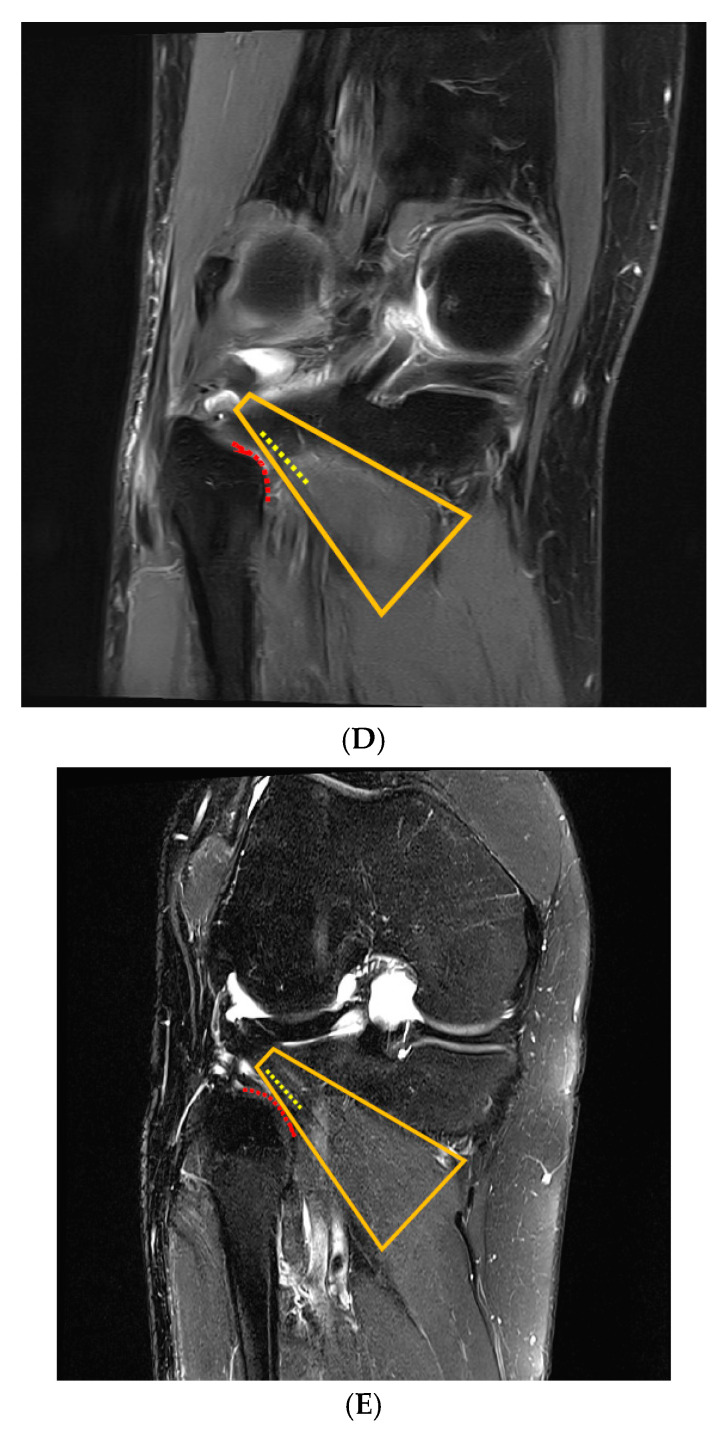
(A) An axial image from the postoperative CT scan. On CT scans with the knee in full extension, the osteotomy plane (yellow dotted box) adjacent to the proximal tibiofibular joint is located on the more medial side parallel to the proximal tibiofibular joint. TSO: tibial-sided osteotomy. (B) On the axial MRI of the knee in full extension, the TSO plane (yellow dotted line), defined on the corresponding postoperative CT scan, is located more medially than the PTFJD plane (red dotted line) and located farther from the peroneal nerve (blue arrow). A line extending from the TSO plane is covered by the popliteus muscle (orange dotted line), whereas the PTFJD is not, indicating that the popliteus muscle does not protect the popliteal neurovascular structures during PTFJD with the knee positioned in full extension. TSO: tibial-sided osteotomy; PTFJD: proximal tibiofibular joint detachment. (C) On the coronal image of the postoperative CT with the knee in full extension, the TSO plane (yellow dotted line) aside the PTFJ (red dotted line) is confirmed. TSO: tibial-side osteotomy; PTFJ: proximal tibiofibular joint. (D) On the coronal MRI of the knee in full extension, the TSO plane (yellow dotted line) defined on the corresponding postoperative CT scan is located more medially compared to the PTFJD plane (red dotted line). The TSO plane is covered by the popliteus muscle (orange line), whereas the PTFJD is not, indicating that the popliteus muscle does not protect the popliteal neurovascular structures during PTFJD with the knee positioned in full extension. TSO: tibial-side osteotomy; PTFJD: proximal tibiofibular joint detachment. (E) Flex 90 MRI. On the coronal MRI of the knee in 90-degree flexion, the TSO plane (yellow dotted line) defined on the corresponding postoperative CT scan is located more medially than the PTFJD plane (red dotted line). The TSO plane is covered by the popliteus muscle (orange line), whereas the PTFJD is not, indicating that the popliteus muscle does not protect the popliteal neurovascular structures during PTFJD with the knee positioned in 90-degree flexion. TSO: tibial-side osteotomy; PTFJD: proximal tibiofibular joint detachment.
